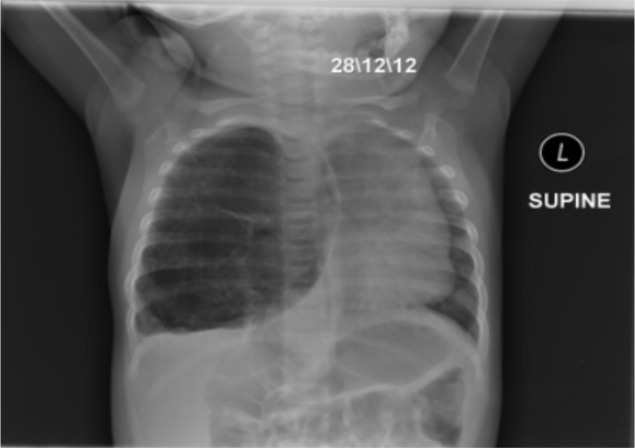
Chest X-ray of an 8-month-old infant with congenital pulmonary malformations N.B: The date on the CXR is according to Ethiopian calendar.
